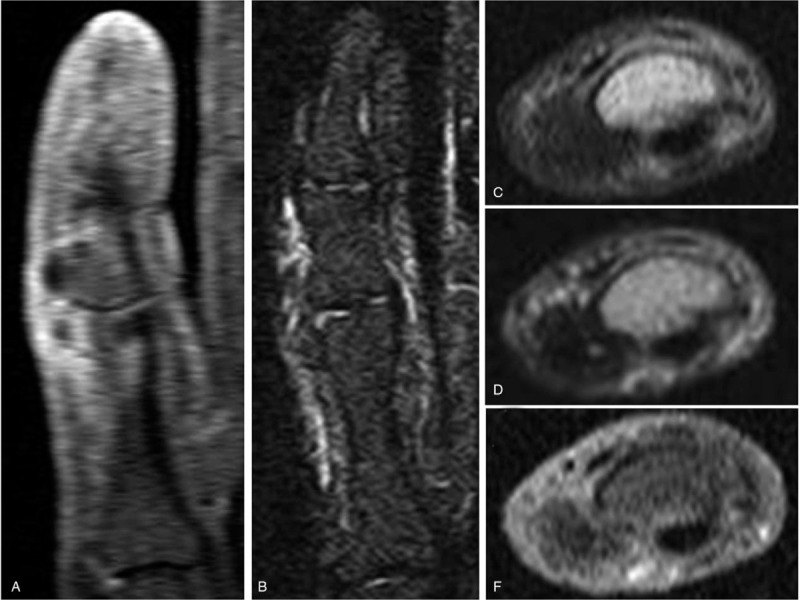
Magnetic resonance imaging of the ulnar side of the proximal and middle fifth phalanges, showing a well-defined humped soft tissue mass with low signal intensity surrounded by a diffuse high signal intensity area on (A) T1-weighted and (B) T2-weighted images. Cross-sectional magnetic resonance imaging of the proximal interphalangeal joint of the left fifth finger, showing that the lesion was well separated from the adjacent bone with no periosteal reaction on (C) T1-weighted, (D) T2-weighted, and (E) contrast-enhanced images.
